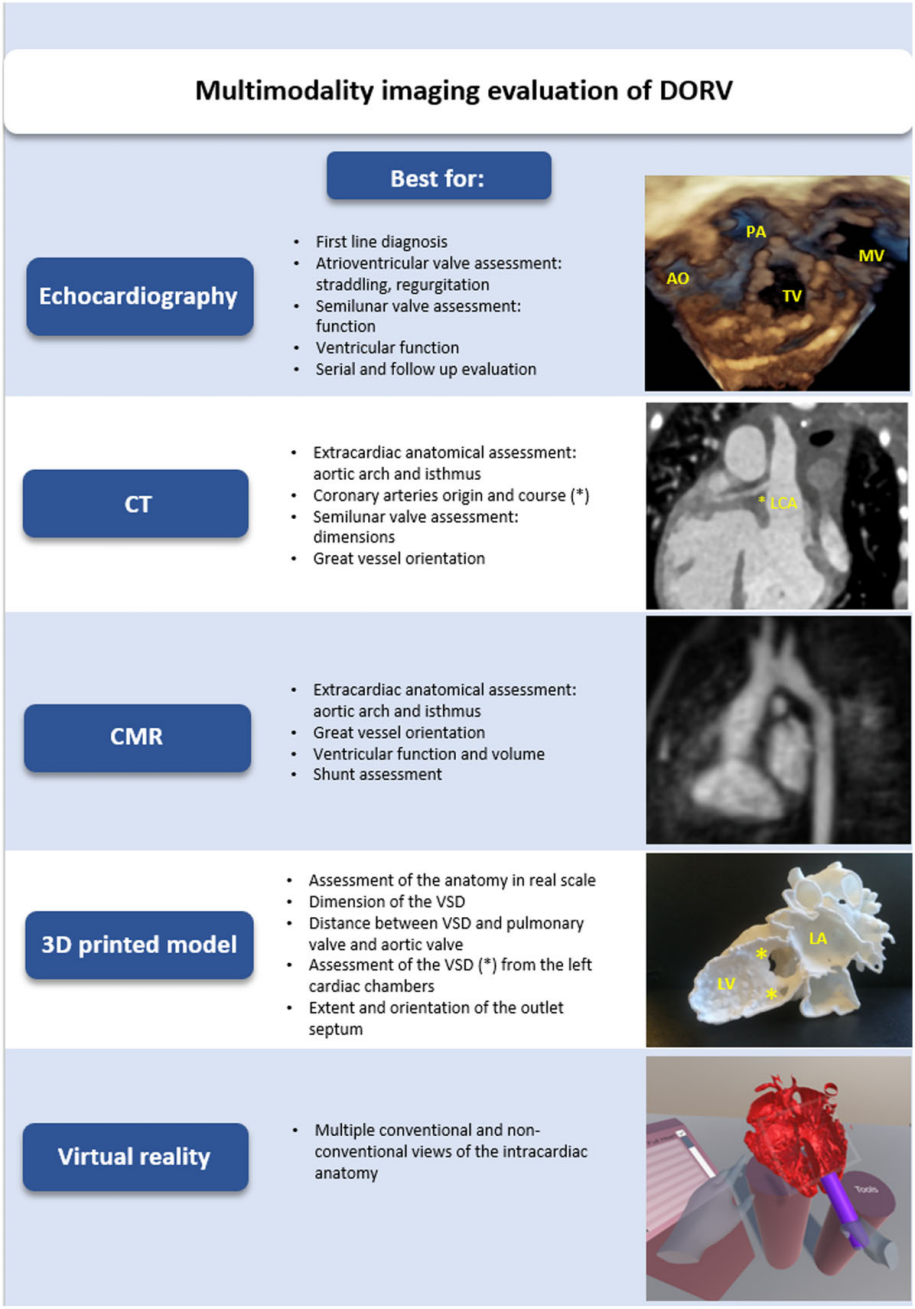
Non-invasive multimodality imaging assessment of double outlet right ventricle: three-dimensional modelling techniques like three-dimensional printing and virtual reality represent additional non-invasive imaging tools for the assessment of complex congenital cases, such as double outlet right ventricle. AO, aorta; CMR, cardiac magnetic resonance; CT, computed tomography; DORV, double outlet right ventricle; MV, mitral valve; PA, pulmoanry valve; TV, tricuspid valve; VSD, ventricular septal defect; 3D, three-dimensional.
